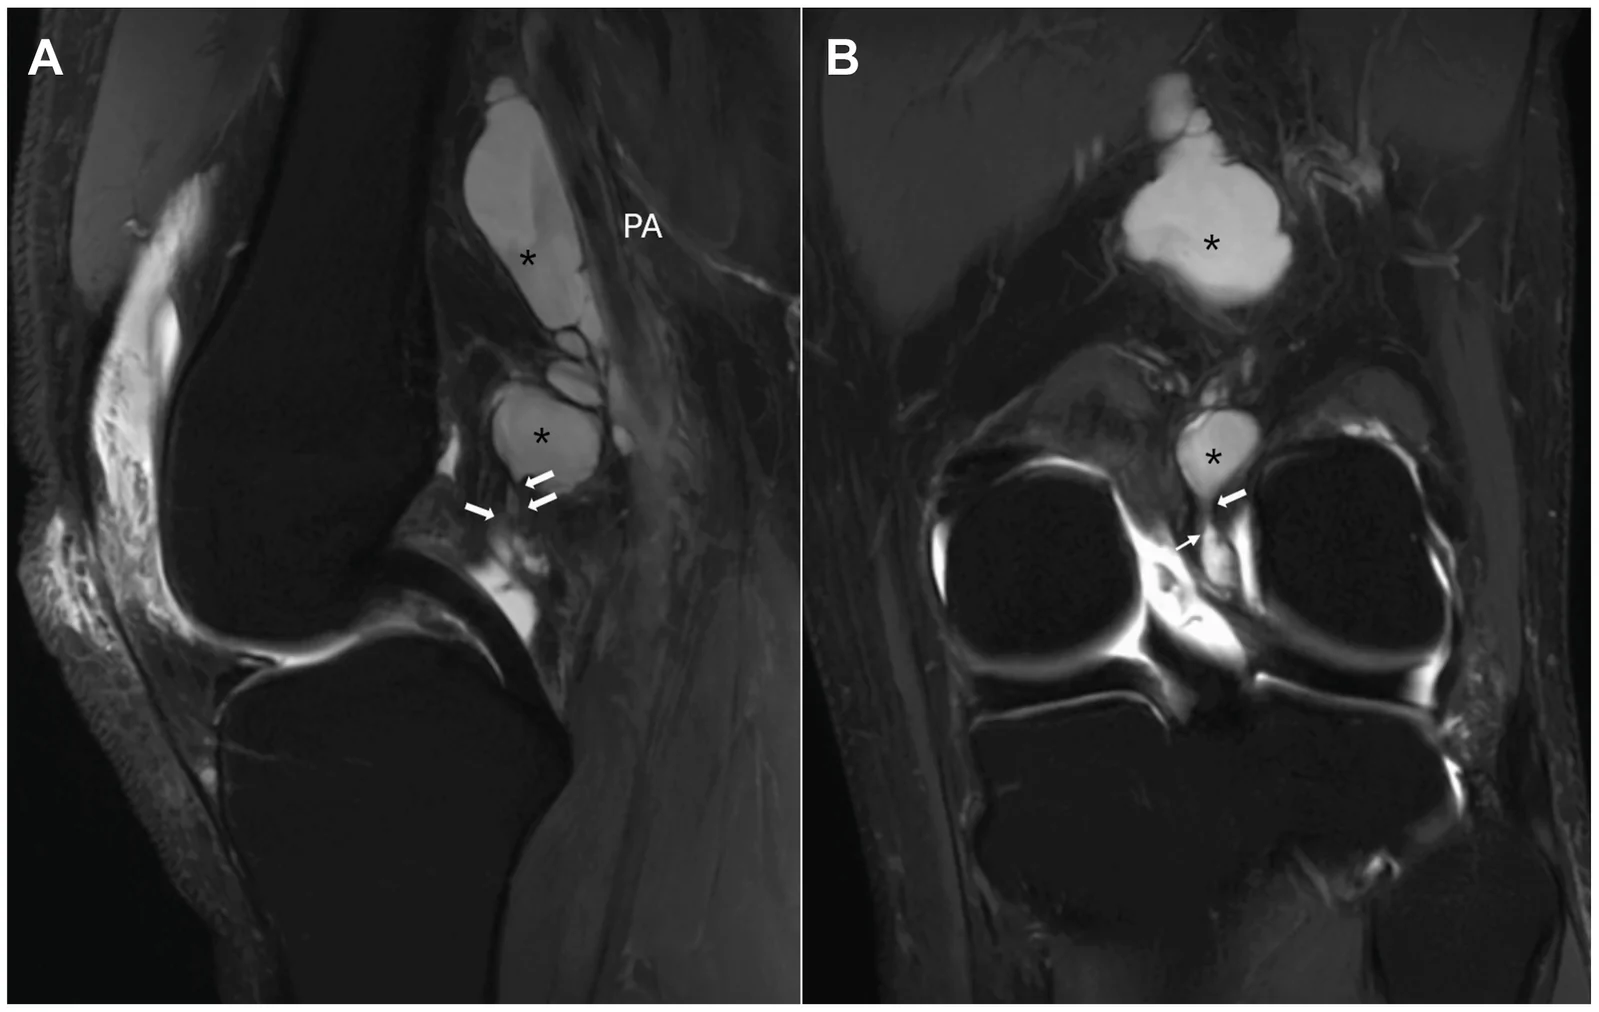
Multiplanar fat-suppressed T2-weighted magnetic resonance (MR) arthrographic images of the left popliteal fossa. A, Sagittal image shows the cyst neck (*bold arrow*). A fluid-fluid level is present within the uppermost cyst component, consistent with gravitational layering of intracystic fluid of differing composition. B, Coronal image shows the cyst neck and its connection to the knee joint (*white arrow*). *∗*, Cyst; *PA*, popliteal artery.
